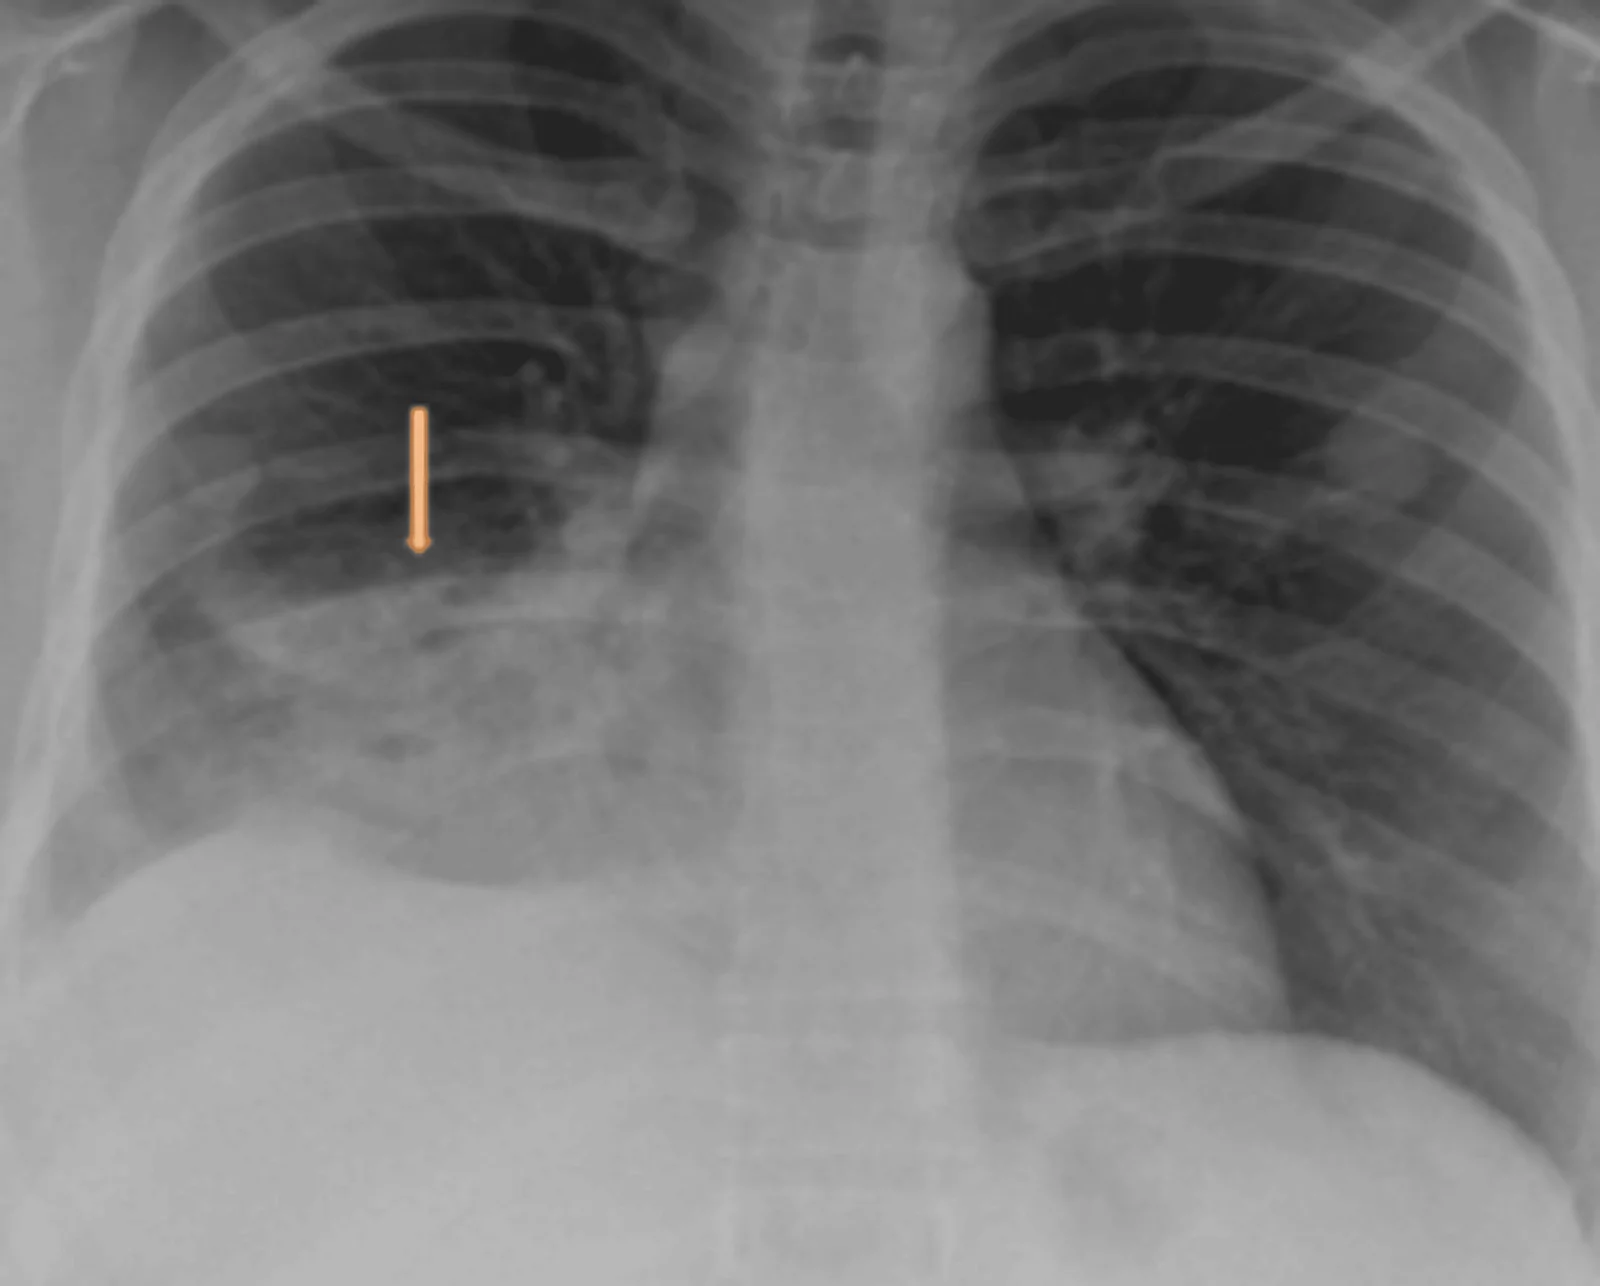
Chest X-ray (on admission) showing the air space opacity concerning for infection The arrow points toward the airspace opacity silhouetting the right heart border in the lower lung zone.
